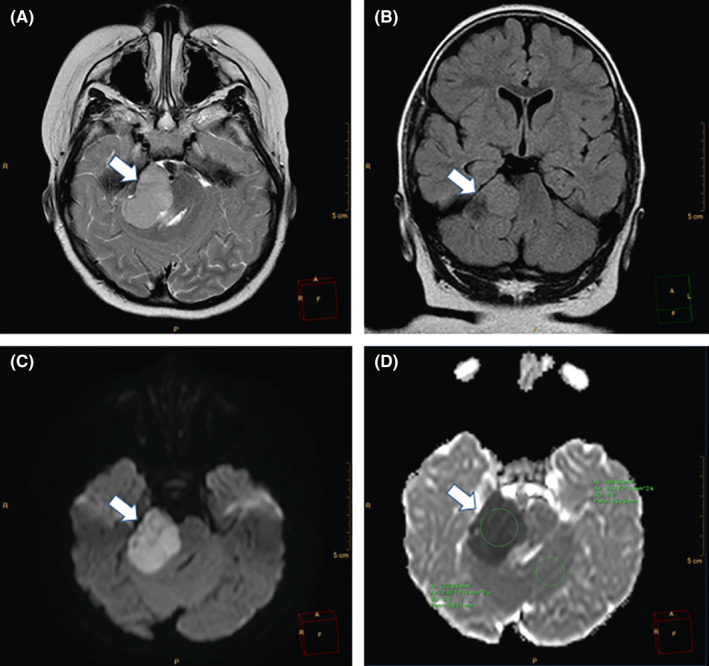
A homogeneously hyperintense solid mass located in the right CPA, on axial T2‐weighted image (arrow, A). The mass appears isointense in coronal FLAIR imaging (arrow, B), hyperintense on axial DWI imaging (arrow, C), and hypointense on the axial ADC map (arrow, D)
